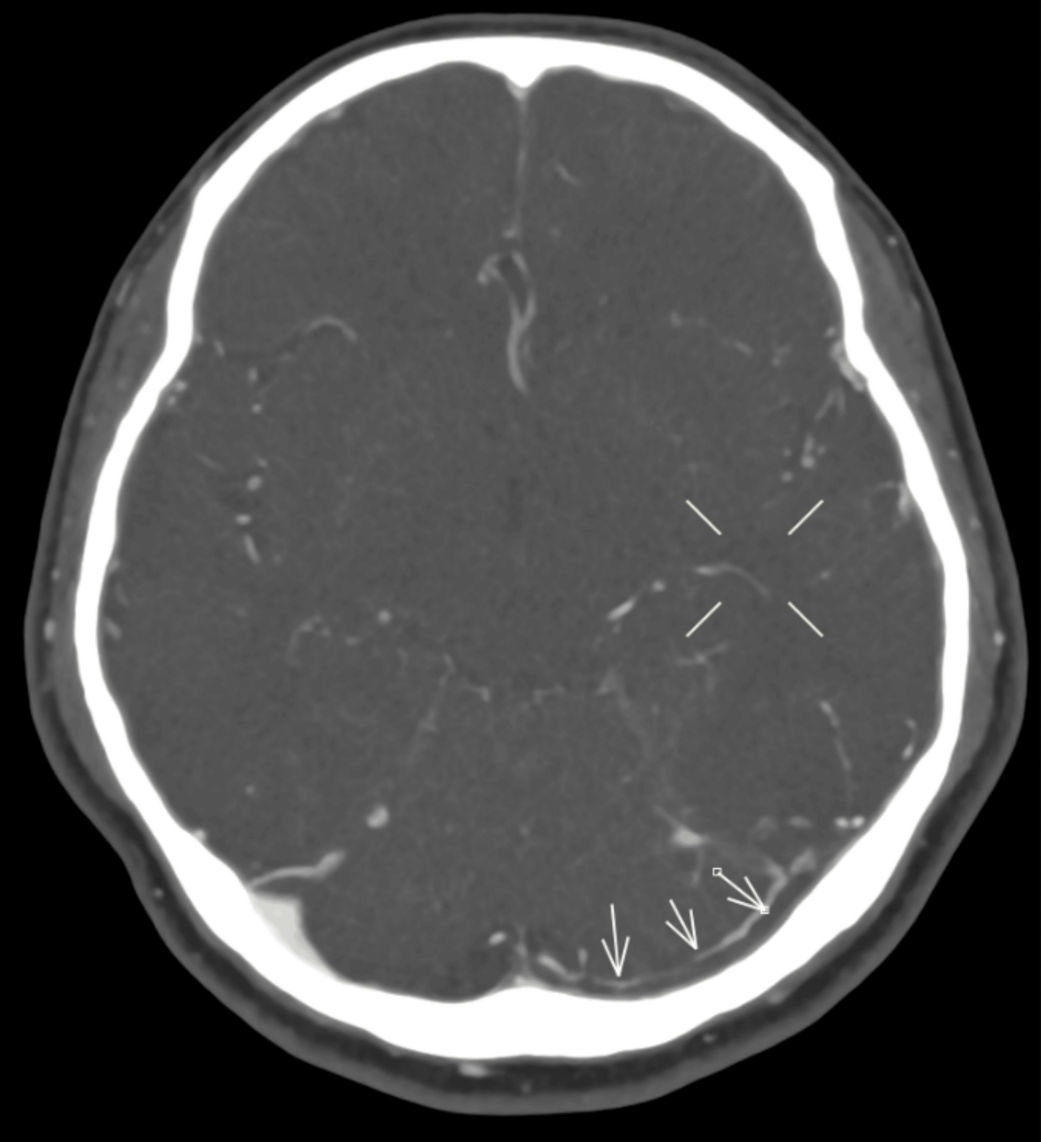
Axial CT venogram demonstrating cerebral venous sinus thrombosis CT venogram demonstrating a filling defect within the left transverse sinus in keeping with cerebral venous sinus thrombosis. CT: computed tomography
